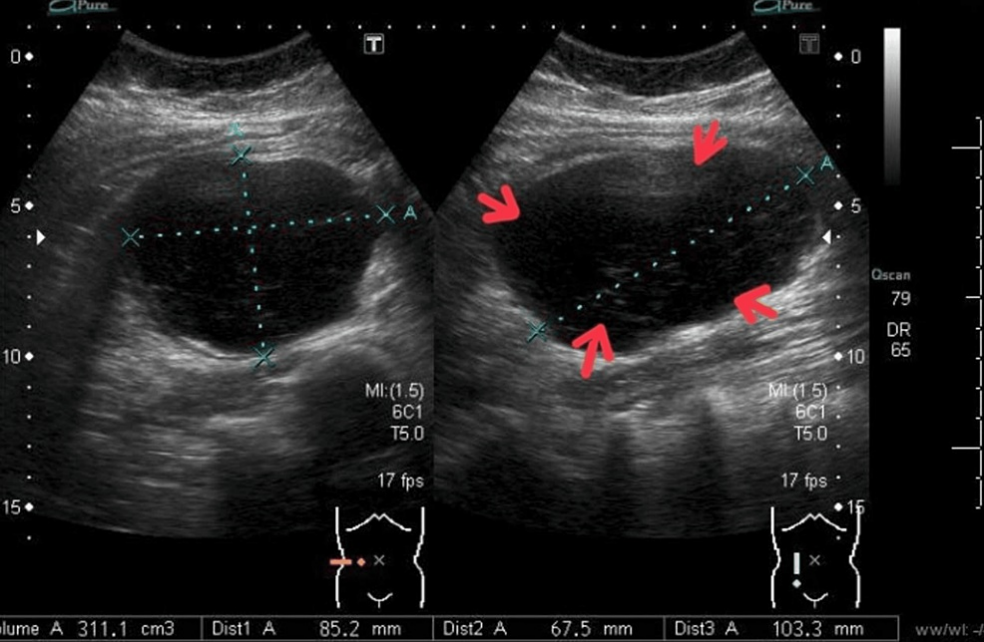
Ascending colon ultrasound revealing a colonic intramural hematoma
